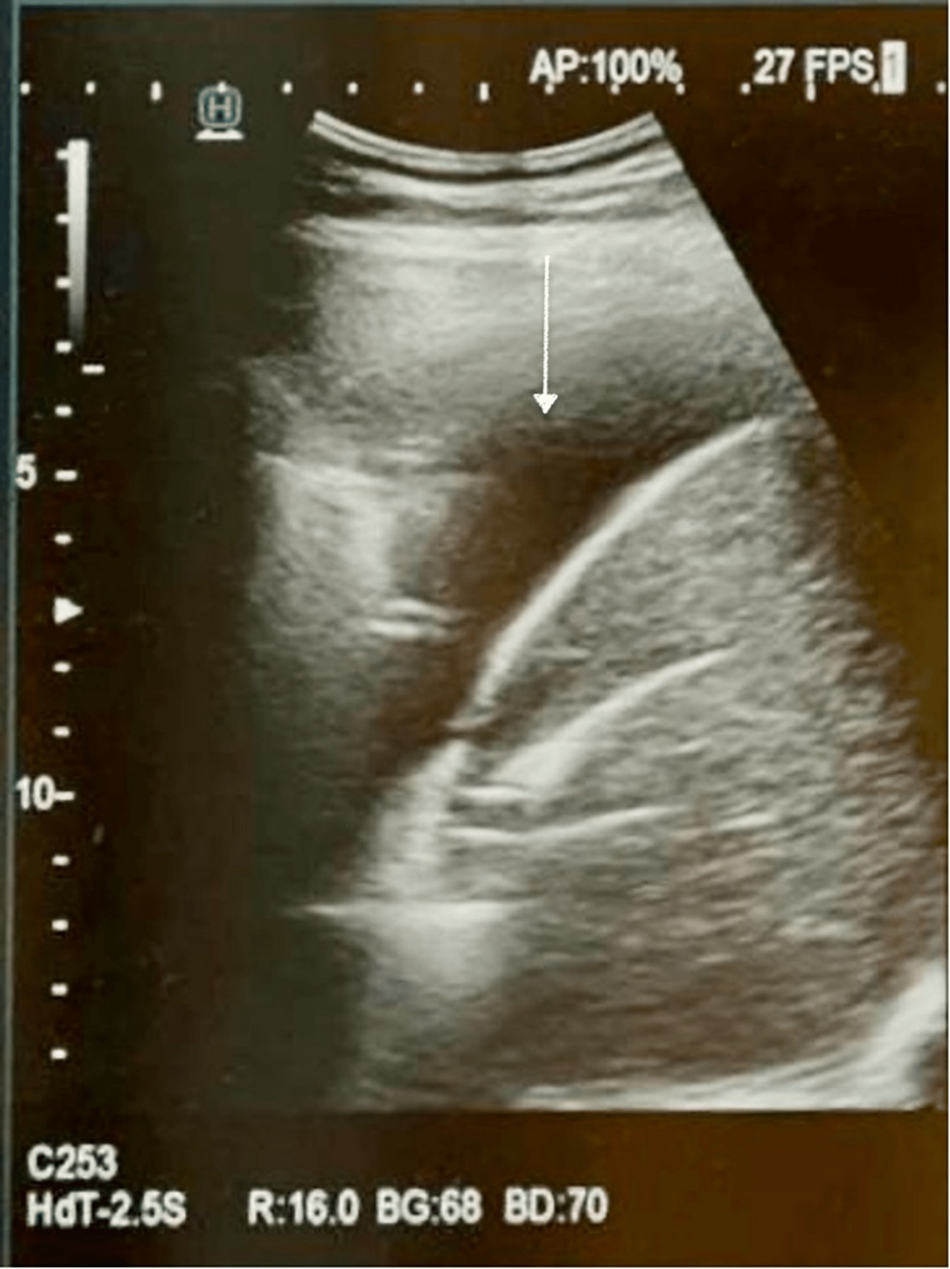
Ultrasonography of the thorax showing pleural effusion with subsegmental lung collapse on the left side (white arrow).
